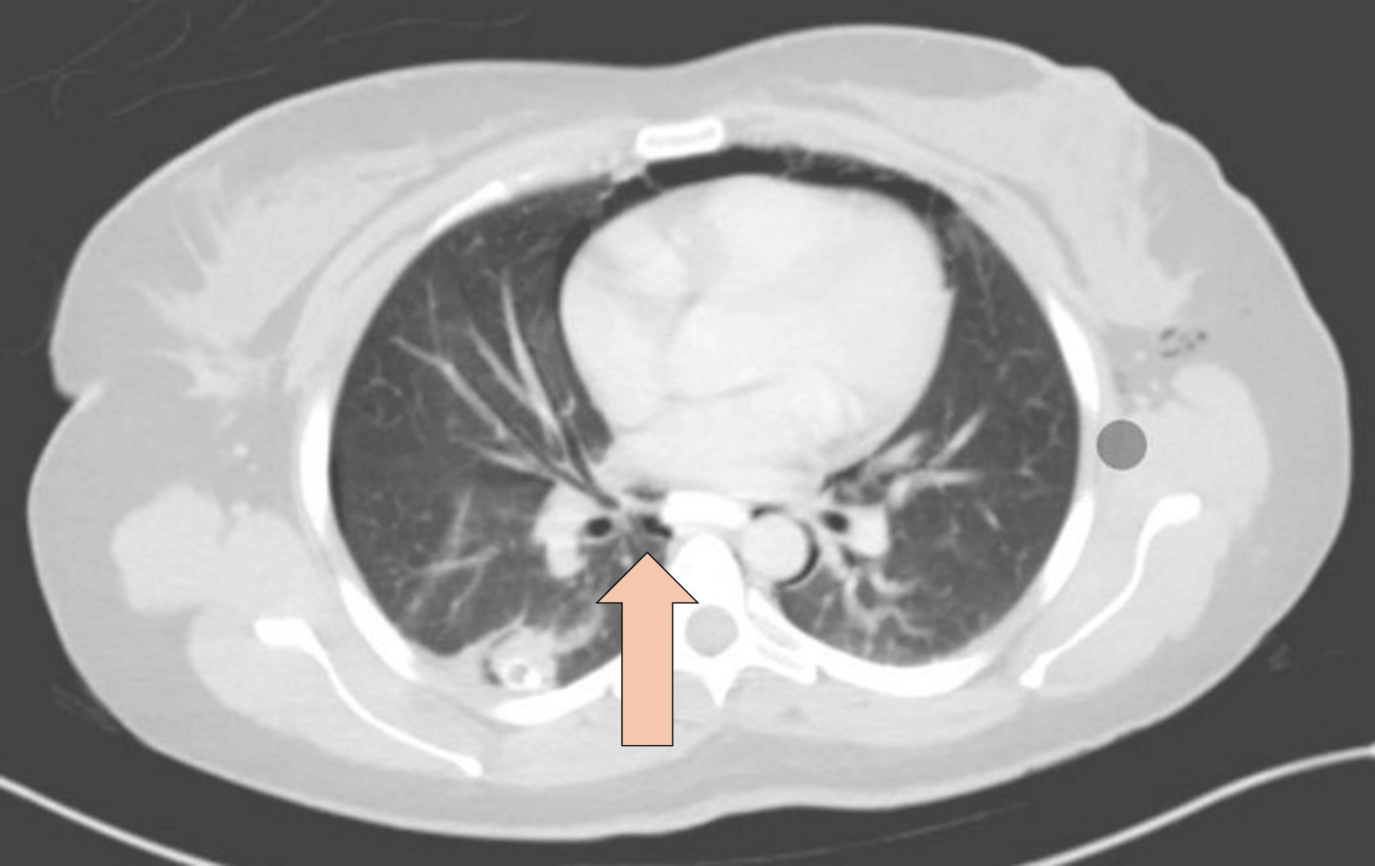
Axial view of CT abdomen with IV and oral contrast post-ERCP showing esophageal perforation The arrow is pointing at the site of esophageal perforation CT: computed tomography; IV: intravenous; ERCP: endoscopic retrograde cholangiopancreatography
